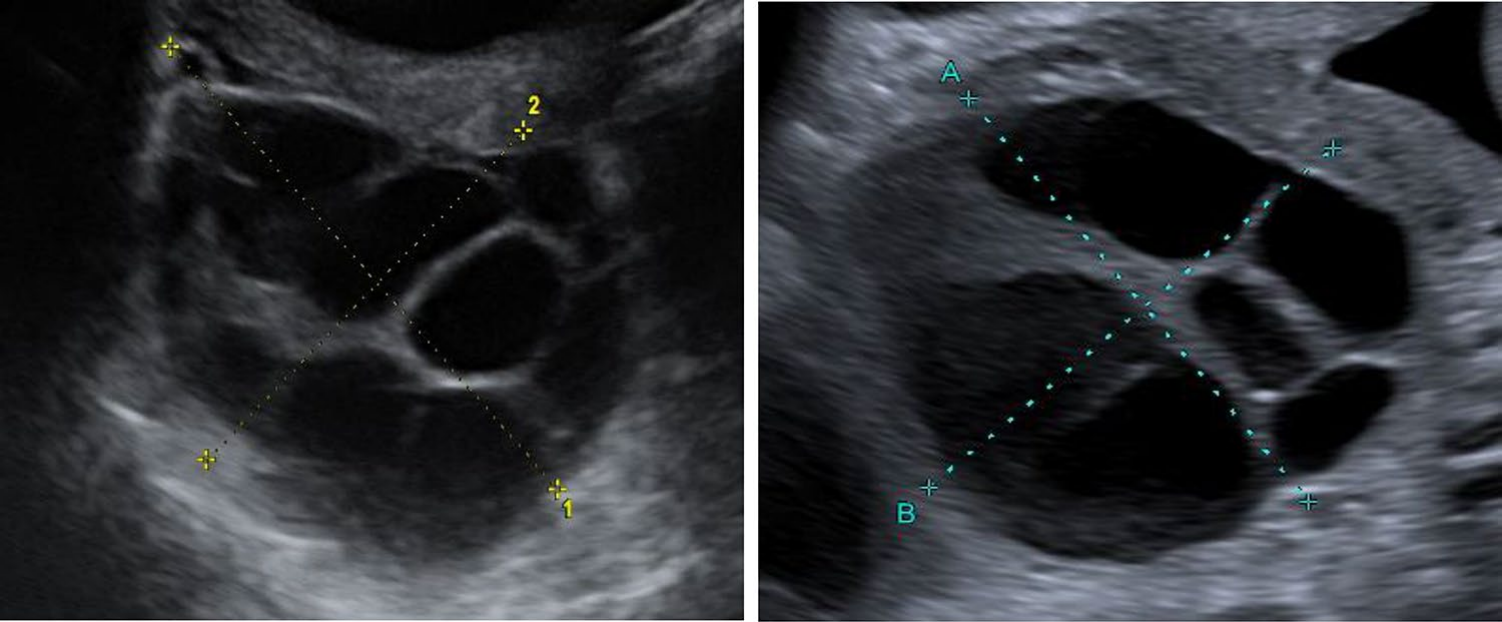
Ultrasound images of the ovaries in a patient with hyperreactio luteinalis at 21 weeks of gestation. a Sagittal plane of the enlarged left ovary (67 × 66 mm); b sagittal plane of the enlarged right ovary (77 × 60 mm)
